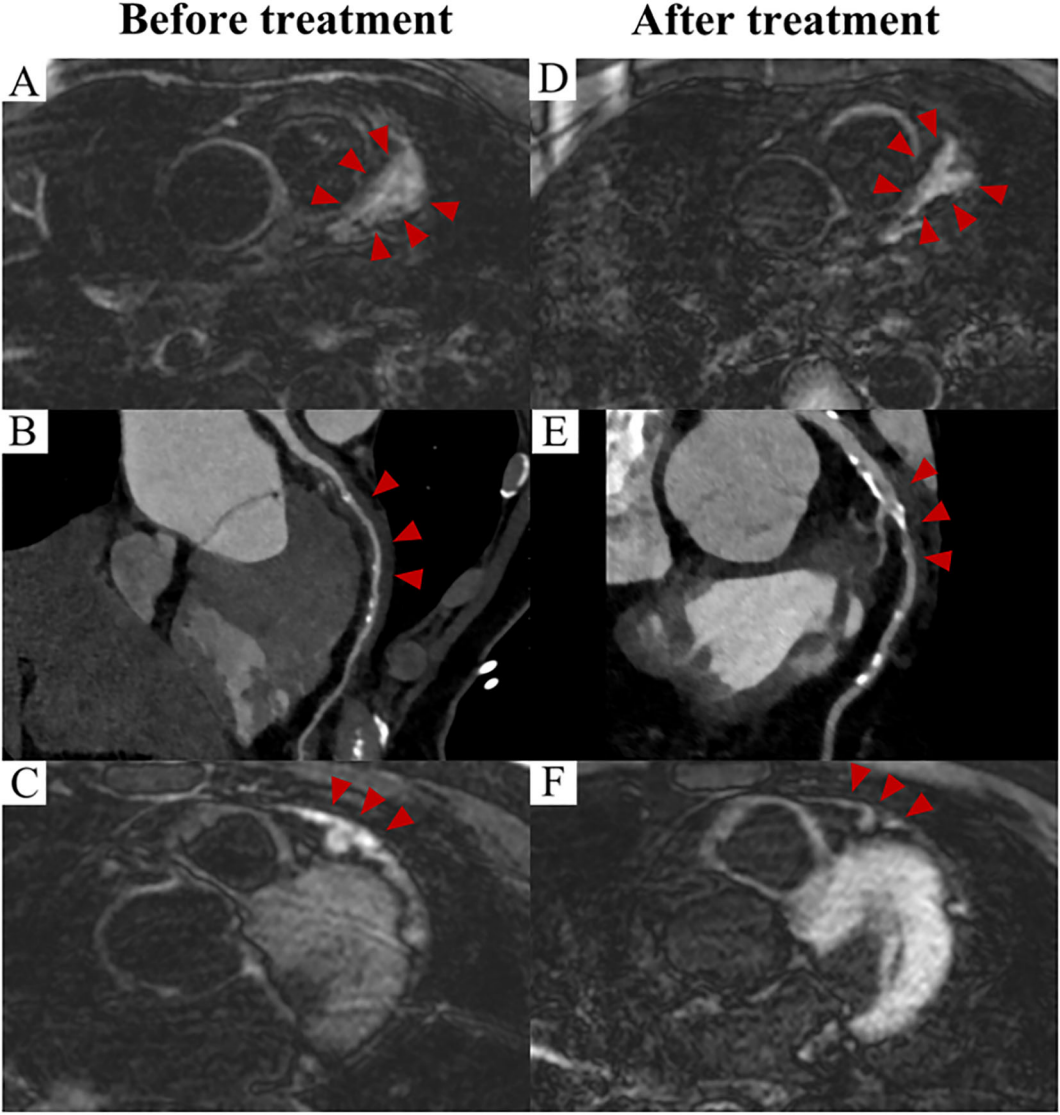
Prognosis of IgG4-related coronary involvement evaluated by imaging (Case 1). CMR image demonstrate wall thickening and enhancement of the LAD before treatment (A), and a reduction in both wall thickening and enhancement were observed after two years of treatment (D). (red arrowheads) CTA images demonstrate the corresponding findings (B, E). (red arrowheads) Pericardial thickening with enhancement was evident on CMR before treatment (C), with no abnormality observed after treatment (F). (red arrowheads). CMR: cardiac magnetic resonance; CTA: computed tomography angiography; LAD: left anterior descending artery.
